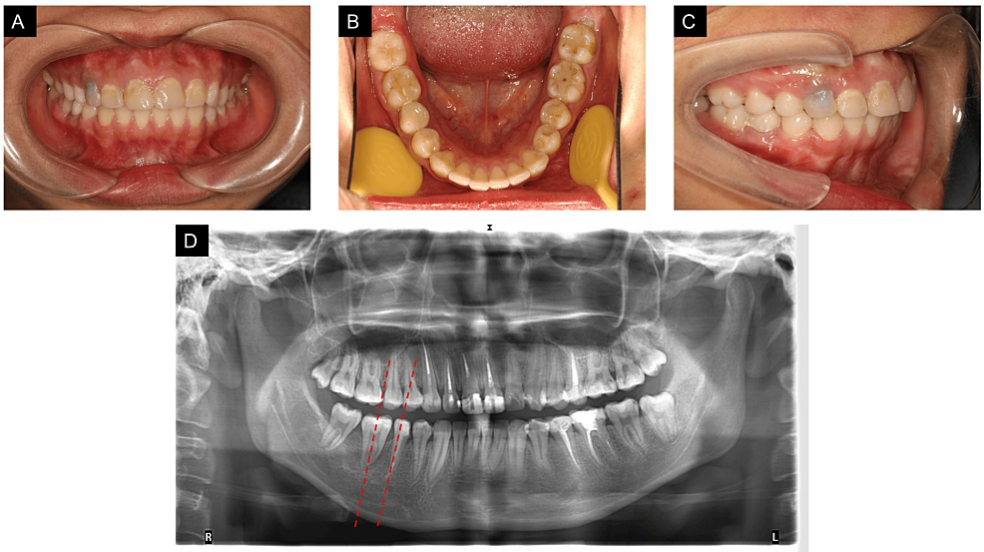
Posttreatment intraoral photographs and posttreatment panoramic radiographs. (A-C) Intraoral photographs. (D) Panoramic radiograph. It was taken before the #45 restoration. The angle between the two red lines was 1.5°.
